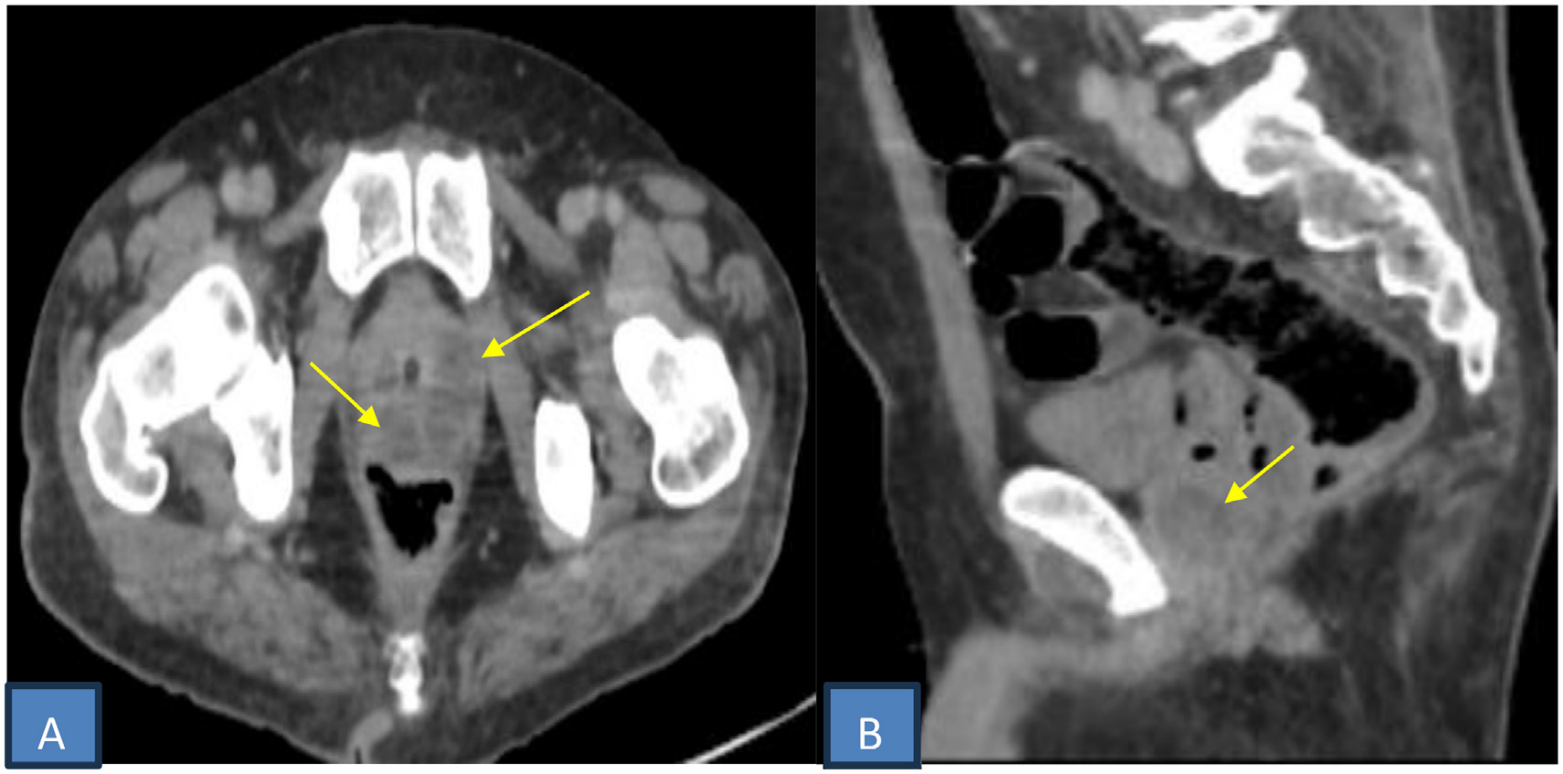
Axial (A) and sagittal (B) pelvic CT images showing two periurethral fluid collections with thick enhancing walls and no internal gas (arrow), consistent with prostatic abscesses.
